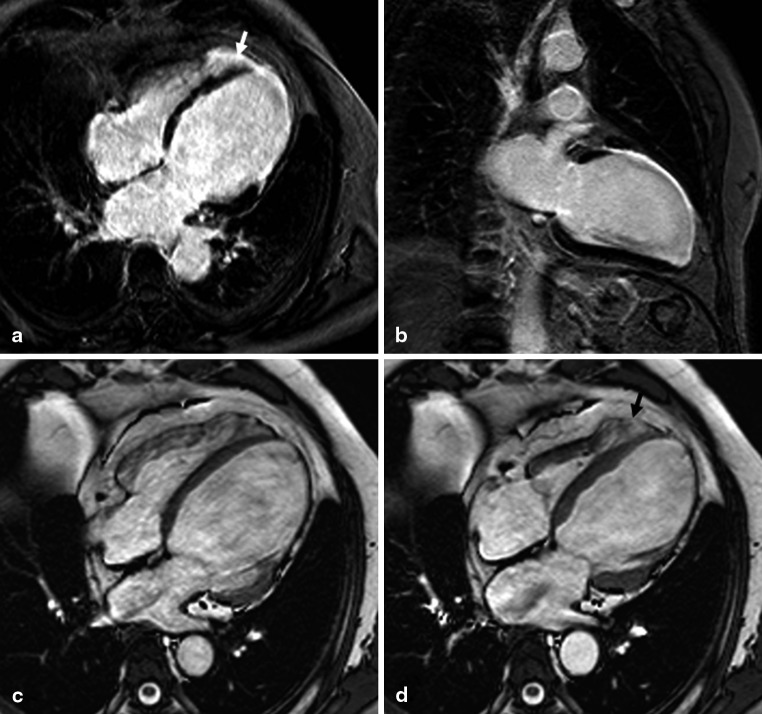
Late gadolinium enhancement (four-chamber view (a), 2 chamber view (b)) and cine (four-chamber view in end-diastole (c) and end-systole (d)) magnetic resonance images of a patient with a large myocardial infarction (left ventricular ejection fraction 22 %) of the left anterior descending coronary artery, continuing from the left ventricular apex to the right ventricle (right ventricular ejection fraction 57 %) (*white arrow*) A corresponding akinetic region of the right ventricular apex can be depicted on the end-systolic cine image (D, *black arrow*)
